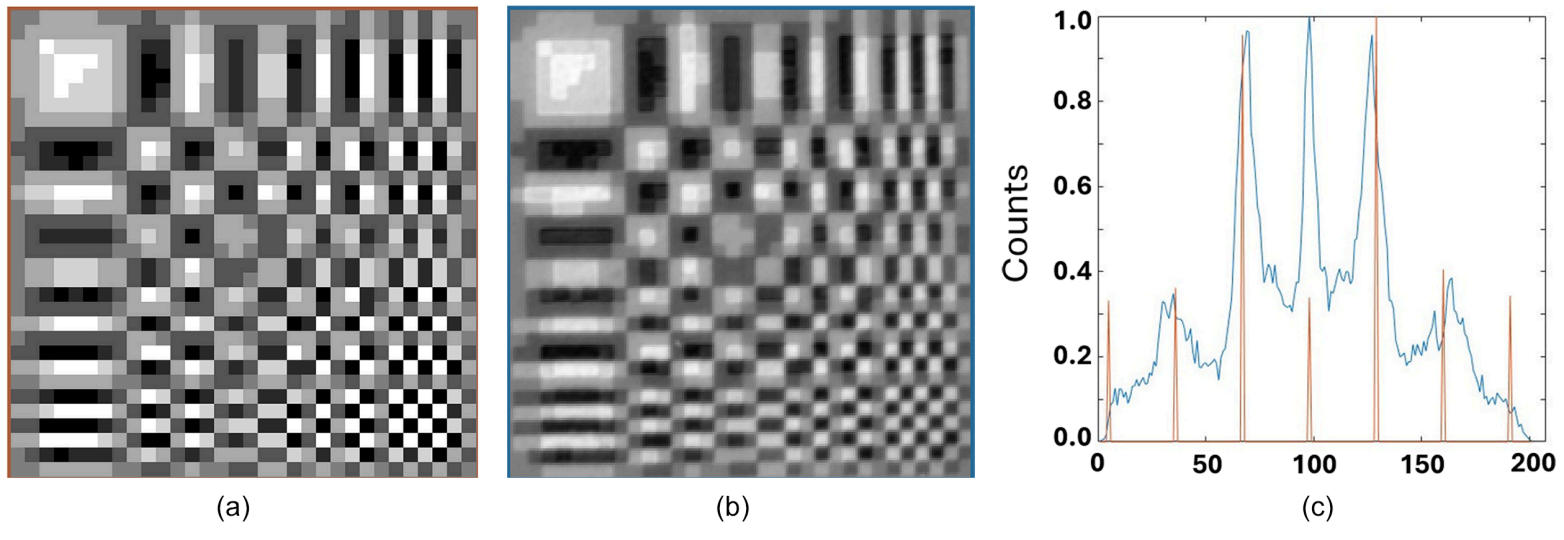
(*a*) Image of an ideal 32 × 32 ± 3 gray-level Huffman-like mask. (*b*) Image of X-ray transmission through the fabricated mask with 20 µm pixel size, presented as *P* − *N*. (*c*) Histogram of graylevels in the ideal mask, image (*a*) (shown in orange), registered with the histogram of (*b*) the intensity of the X-rays transmitted through the fabricated mask (shown in blue). Both image histograms show seven closely matched peaks, representing the relative X-ray transmission intensities for mask levels −3, −2, −1, 0, +1, +2, +3. The vertical scale is counts; the horizontal axis is relative intensity.
